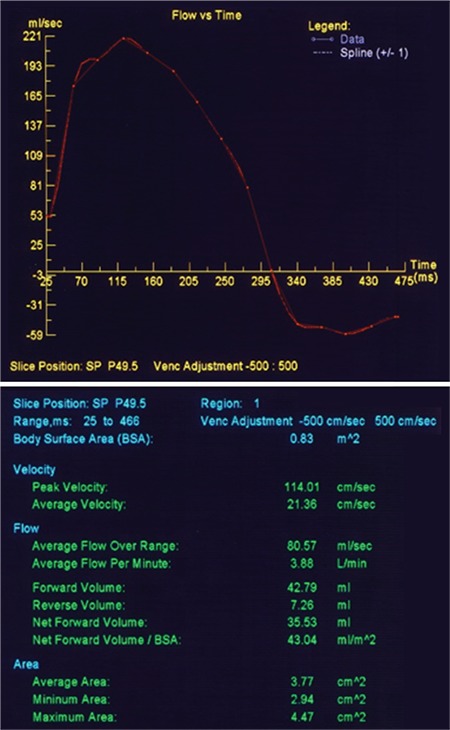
MRI quantification of pulmonary regurgitation. In this patient the regurgitation fraction was calculated as 17% by flow analysis.
